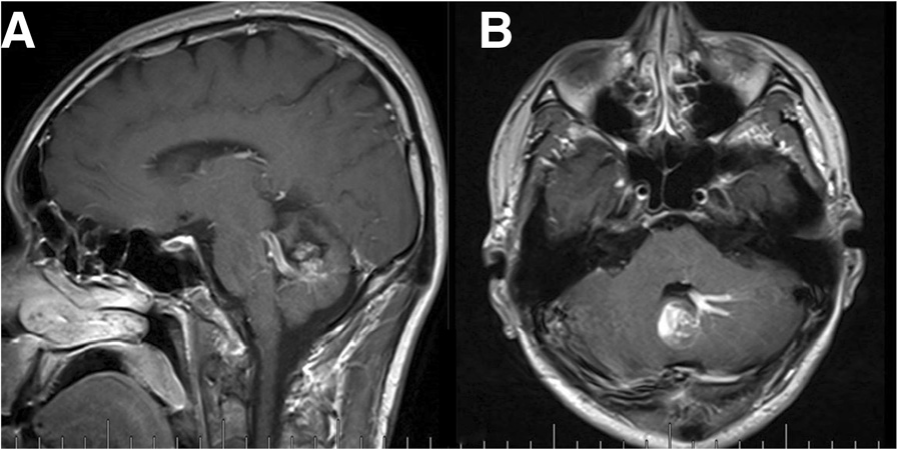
Subtentorial DVA and Concomitant CM. A and B. Enhanced MRI images showing irregular “caput medusae” in the left cerebellar hemisphere and pons, and the CM locates at the distal radicles of the DVA.
